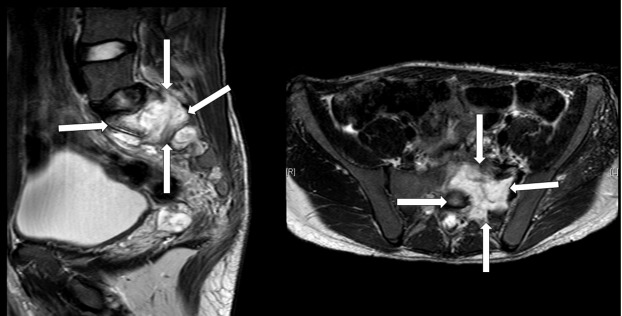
A preoperative T2-weighted MRI scan showing a tumor mass invading the sacral bone (white arrows).
